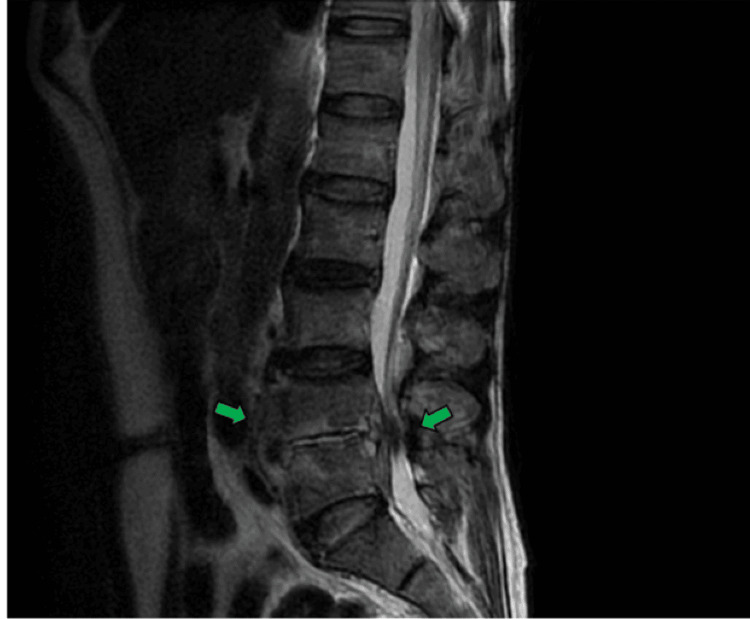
Contrast-enhanced MRI of the lumbar spine, sagittal view, T2 FRFSE sequence. The green arrows indicate signs of spondylodiscitis at the L4-L5 level, showing bone edema of the adjacent vertebral bodies and left paravertebral inflammatory involvement. T2 FRFSE, T2 fast recovery fast spin echo.
